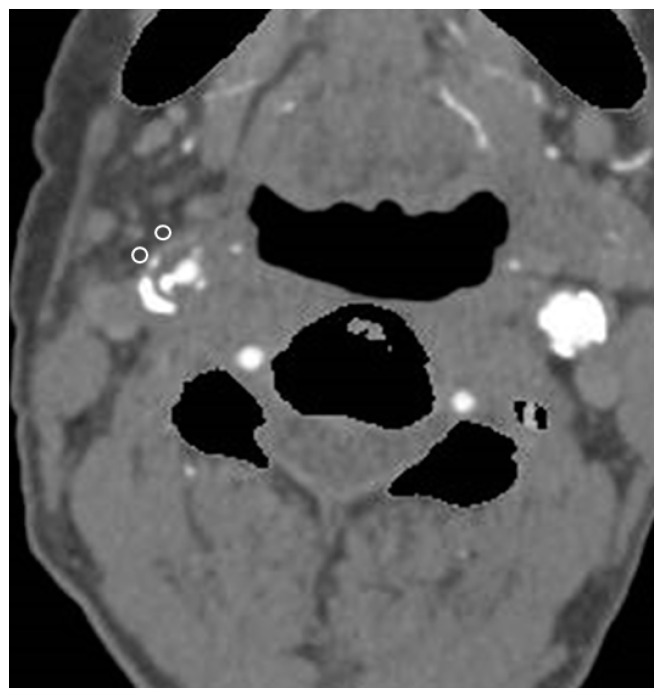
Computed tomography angiography of a 76-year-old man showing right carotid stenosis. Two regions of interest ROIs (white, round) were placed in the pericarotid fat around the carotid plaque. In this case, the 2 ROIs were measured as −55.3 and −58.2.
